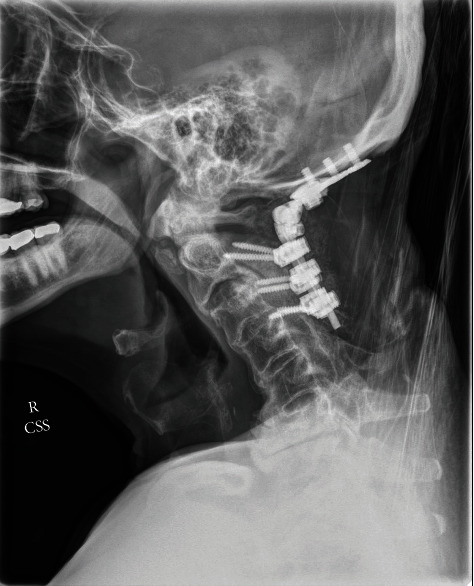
Postoperative lateral cervical spine X-ray demonstrates occipitocervical fusion with a C2-C4 lateral mass screw and posterior rod construct with the occipital plate.
